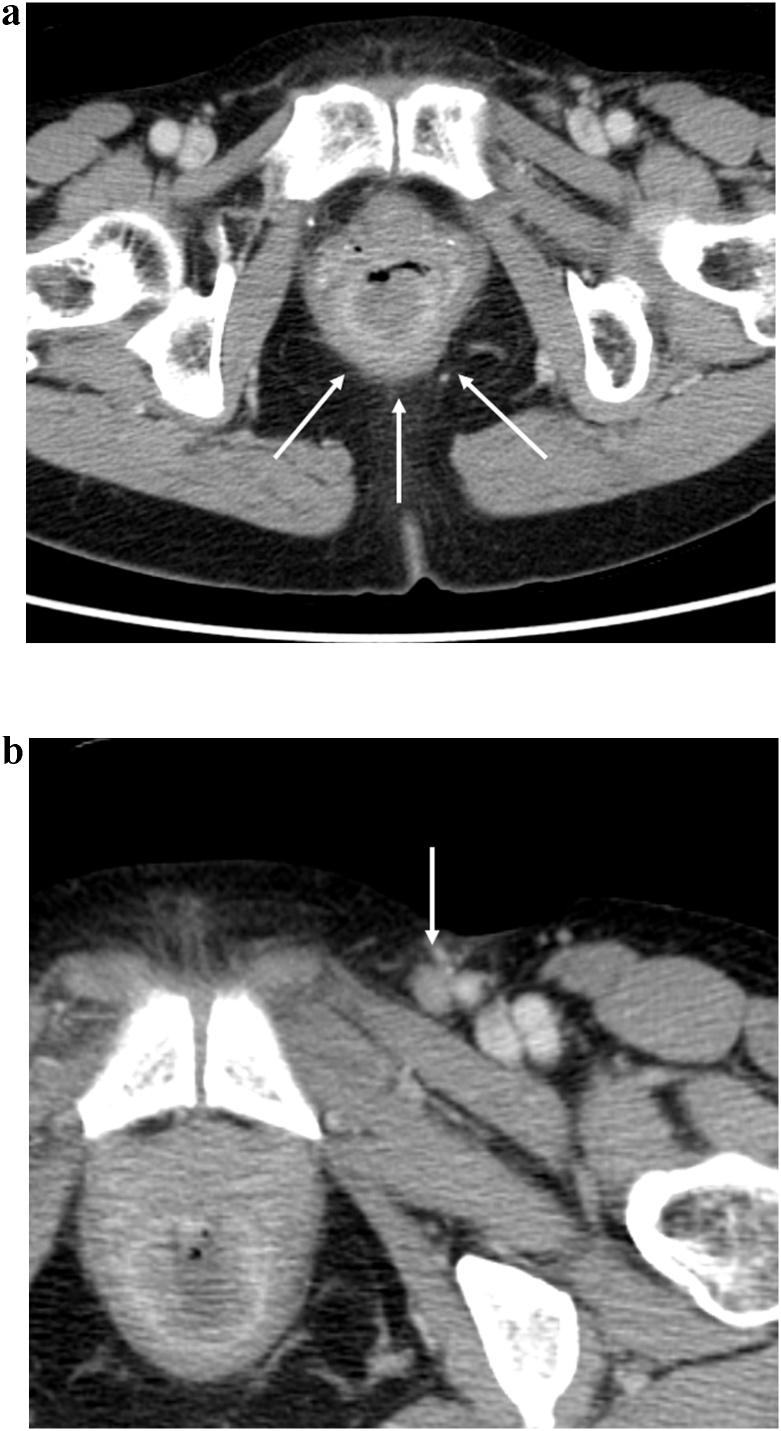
Abdominal contrast-enhanced CT findings. a: The mass with enhancement detected at the anal canal　(white arrow). b: An enlarged left inguinal lymph node (white arrow).
